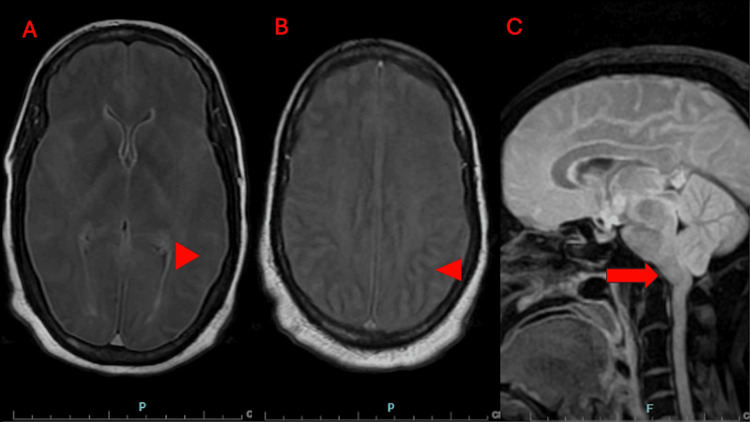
MRI of the brain without contrast. (A, B) Caudal to cranial sequence demonstrating diffuse abnormal signal throughout and sulcal effacement (arrowhead). (C) Mild transtentorial and cerebellar tonsillar herniation (arrow).
